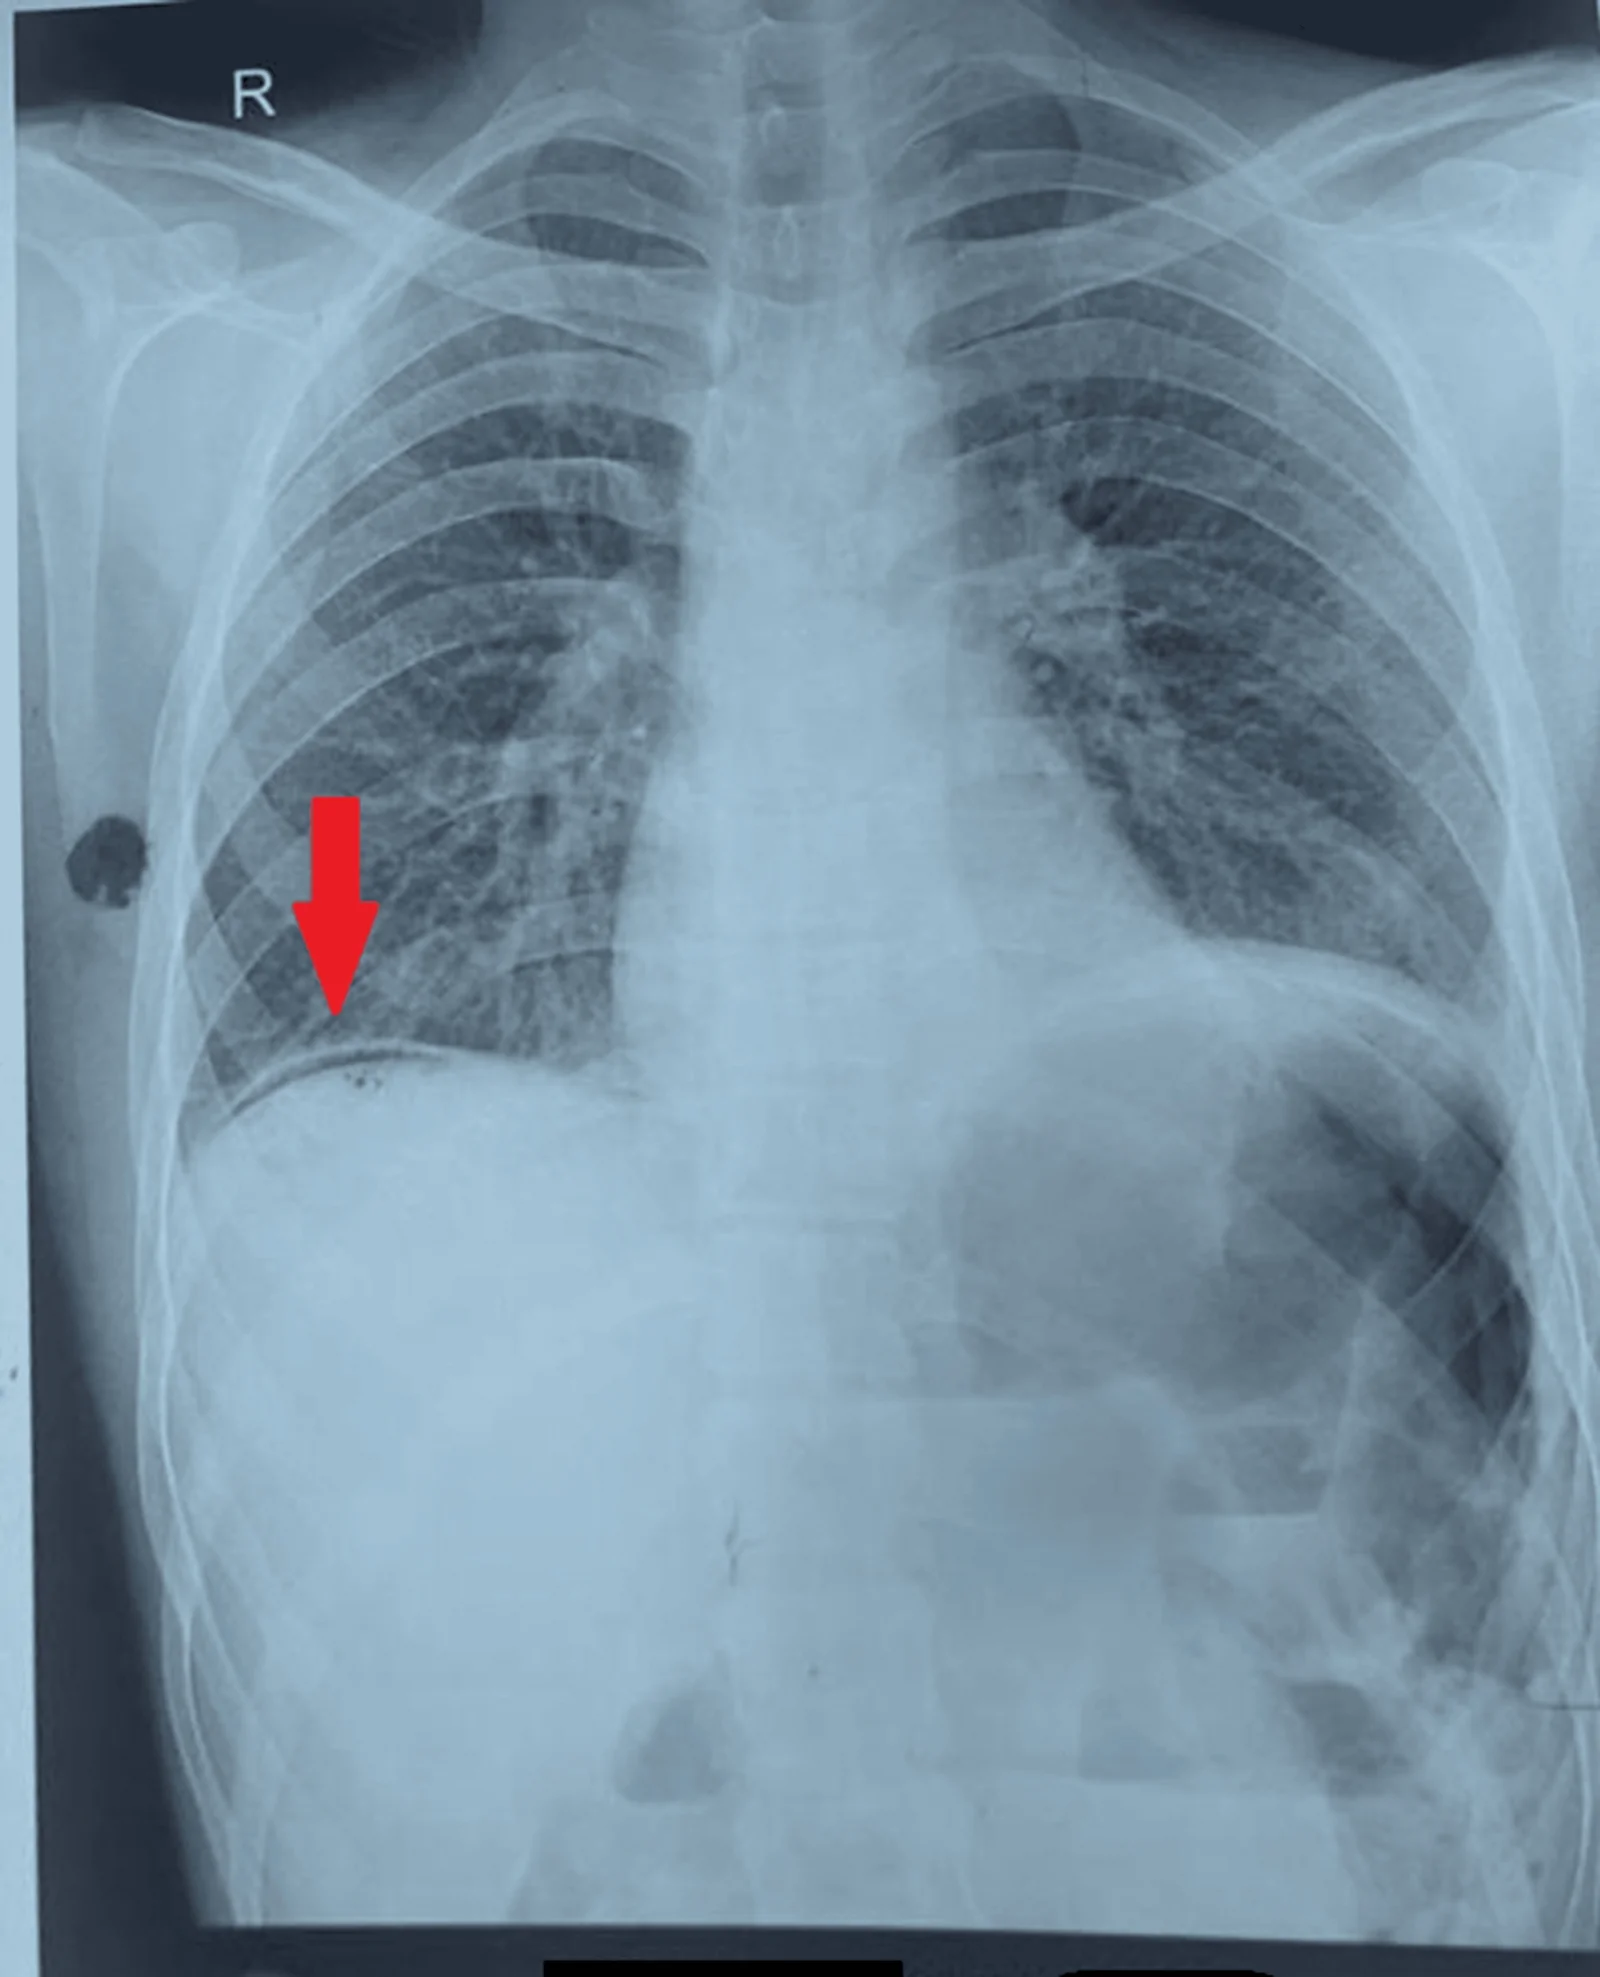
Chest X-ray (posteroanterior (PA) view) showing gas under the diaphragm (red arrow).
